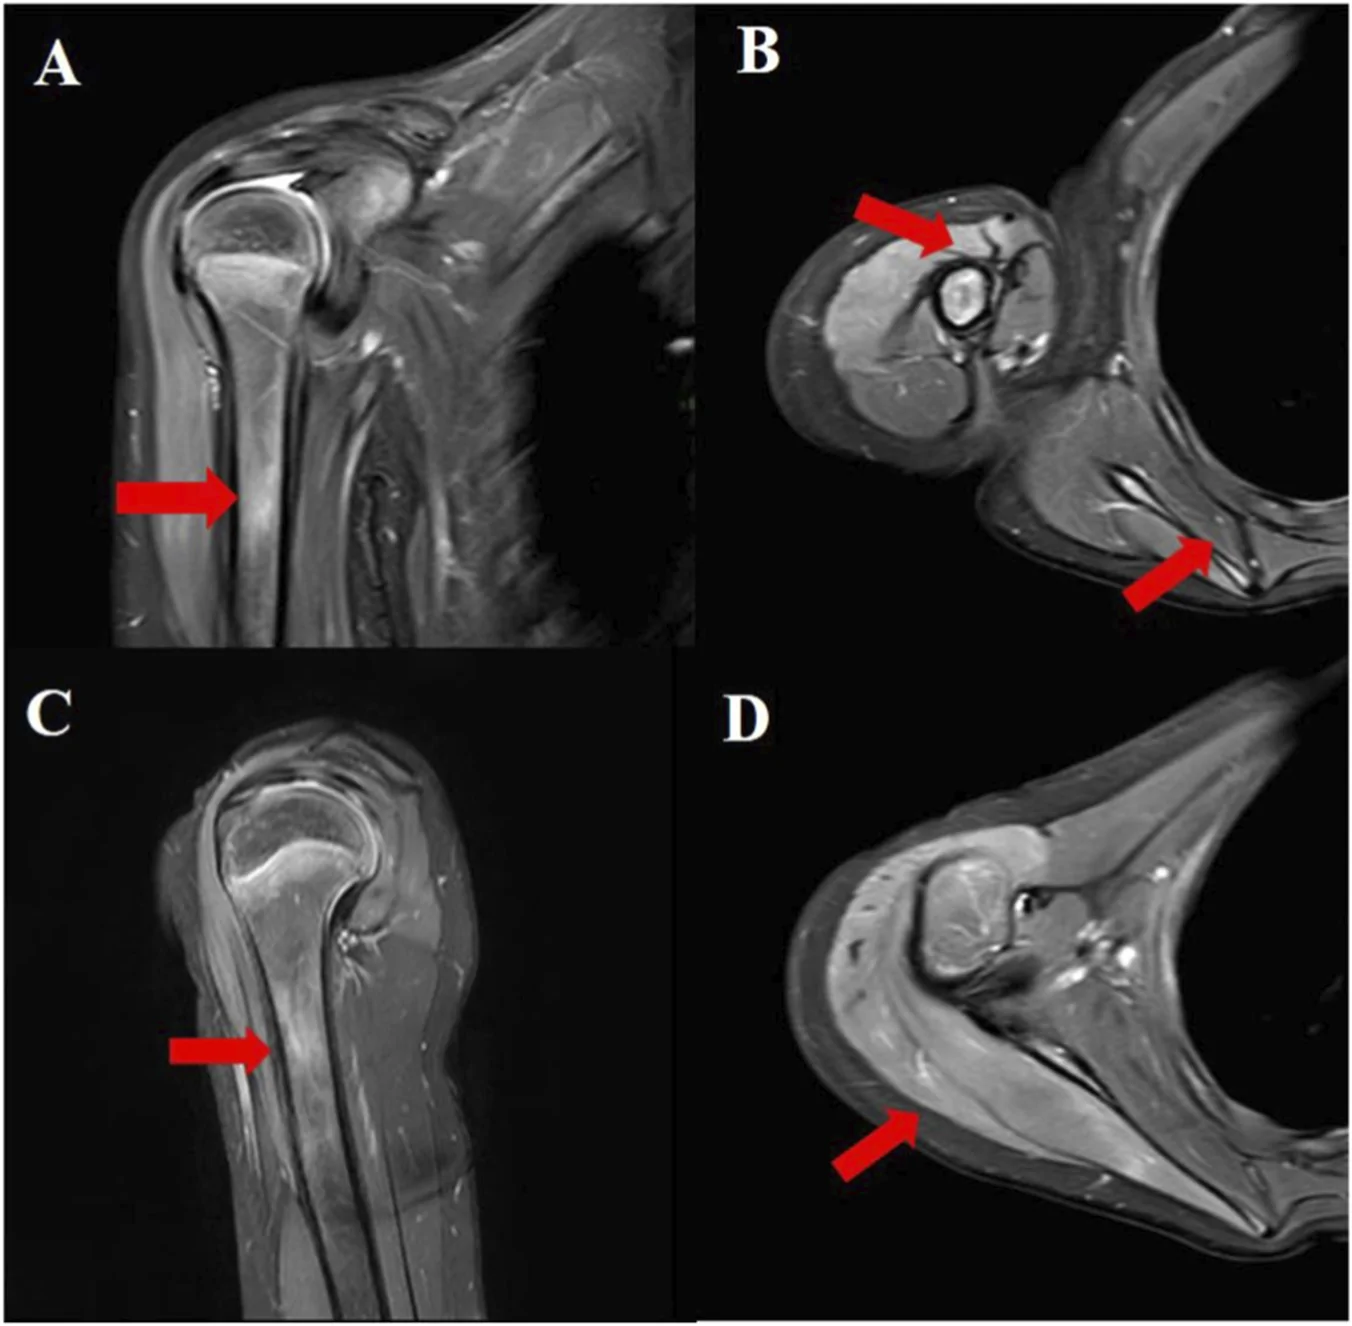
Magnetic resonance imaging (MRI) of the right shoulder and brachial plexus at initial presentation. (A) Coronal T2-weighted fat-suppressed image showing hyperintense signal (arrow) within the proximal right humeral diaphysis and metaphysis, consistent with bone marrow edema. (B) Axial T2-weighted fat-suppressed image demonstrating mild joint effusion (upper arrow) and edema of the surrounding musculature (lower arrow), including the deltoid and rotator cuff muscles. (C) Sagittal T2-weighted fat-suppressed image revealing hyperintense signal (arrow) in the proximal humeral metaphysis, suggestive of bone marrow edema extending along the humeral shaft. (D) Axial T2-weighted fat-suppressed image at a different level showing soft tissue edema (arrow) surrounding the right shoulder joint, with involvement of the subcutaneous tissues and adjacent musculature.
